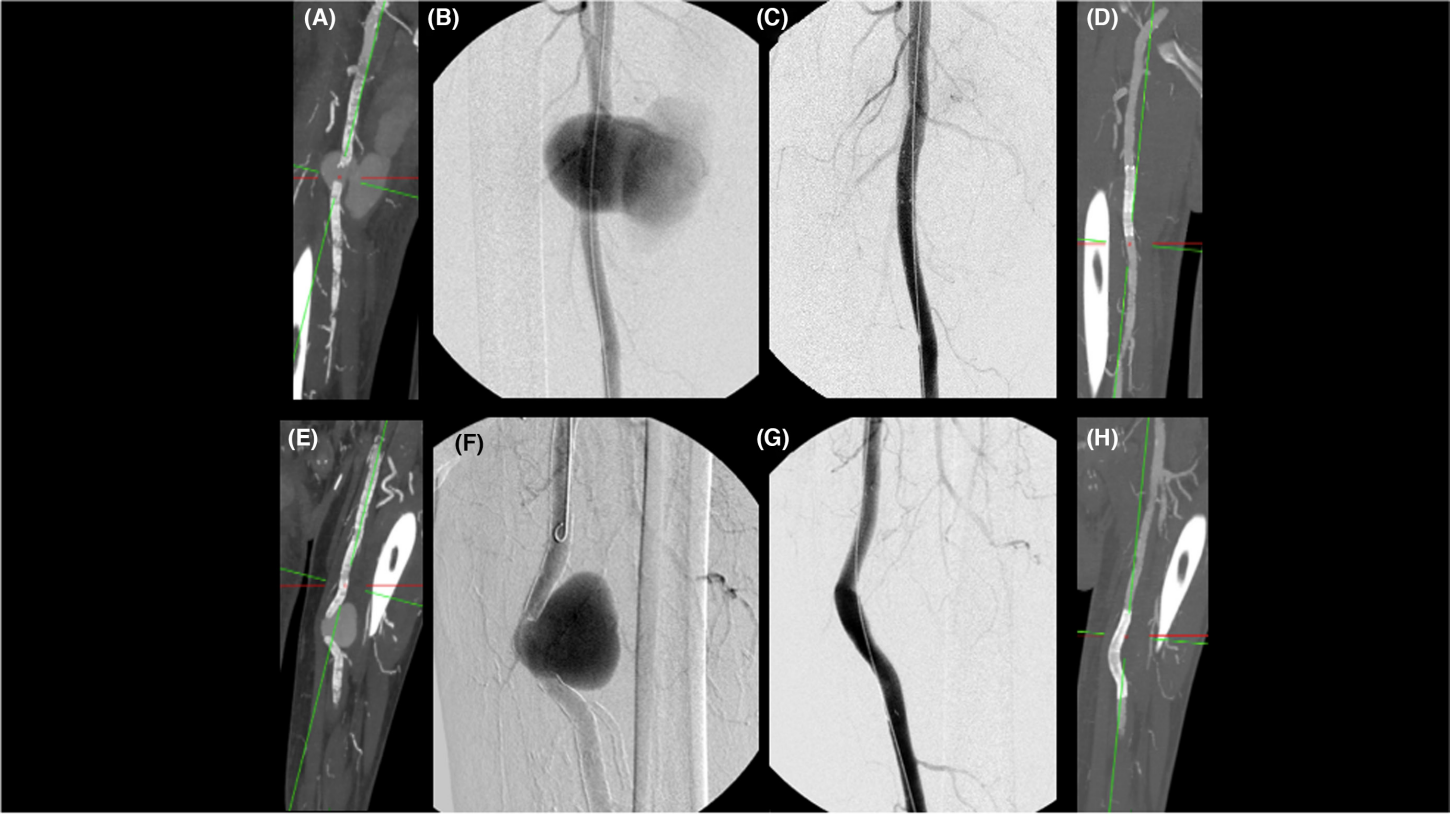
Preoperative computed tomography (CT) angiographic (A) and intraoperative digital subtraction angiographic (B) images demonstrating the ruptured right superficial femoral artery pseudoaneurysm. Intraoperative digital subtraction angiographic (C) and postoperative CT angiographic (D) images demonstrating Viabahn stent graft exclusion of the ruptured right superficial femoral artery pseudoaneurysm. Preoperative CT angiographic (E) and intraoperative digital subtraction angiographic (F) images demonstrating the left superficial femoral artery pseudoaneurysm. Intraoperative digital subtraction angiographic (G) and postoperative CT angiographic (H) images demonstrating Viabahn stent graft exclusion of the left superficial femoral artery pseudoaneurysm.
